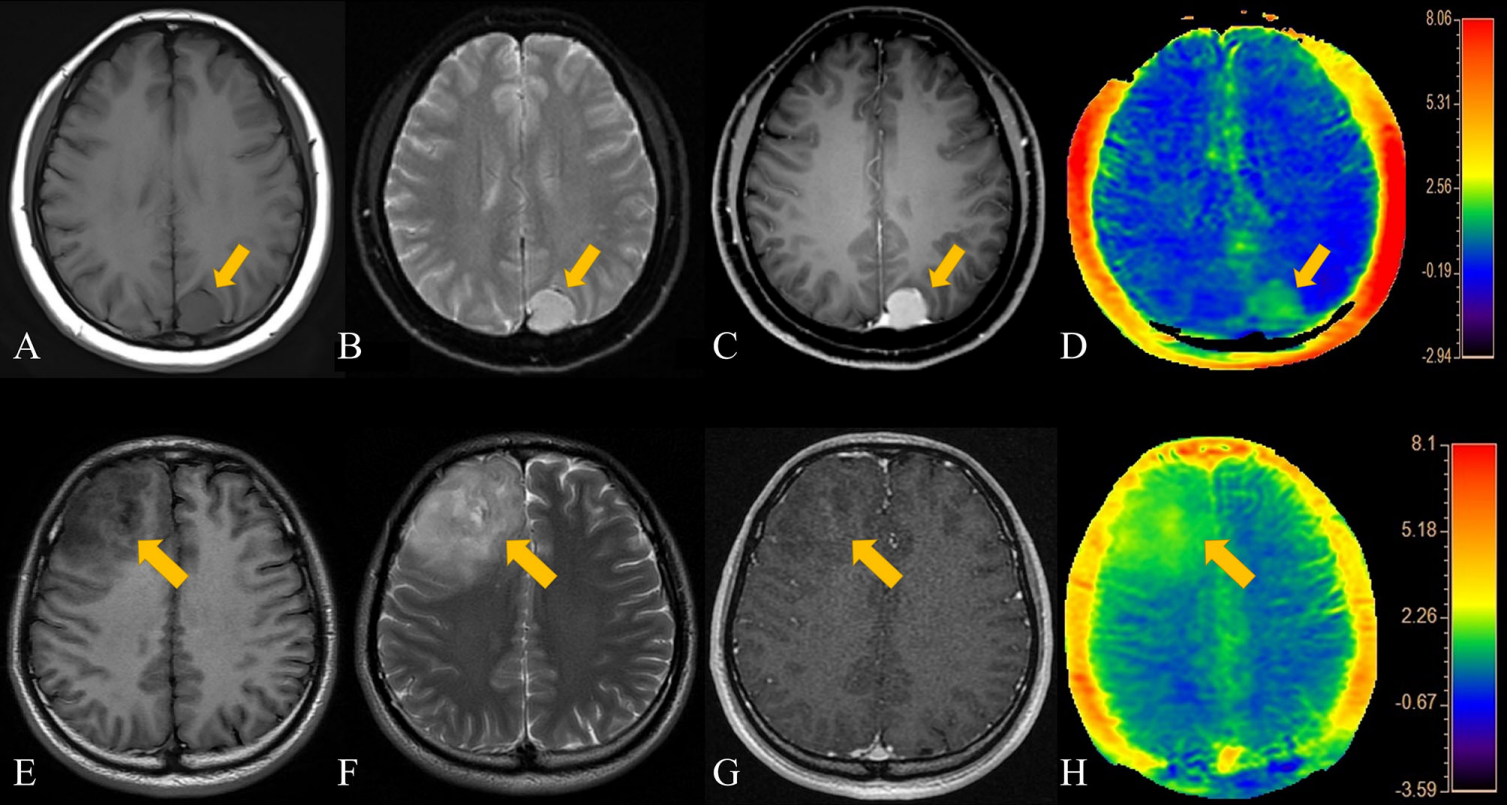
Patient 1, a 44-year-old female, presented with a left parietal lobe meningioma. The patient's T2WI, contrast-enhanced MRI, and APT range were similar. (A) T1-weighted imaging showed an iso-slightly low signal. (B) T2-weighted imaging showed an iso-slightly high signal. (C) Enhanced MR imaging showed marked enhancement. (D) APT imaging shows a slightly higher rate of amide proton transfer. Patient 2, a 34-year- old male, had an LGG in the right frontal lobe. The APT signal range of the patient was slightly larger than that on both the T2WI and enhanced scans. (E) T1-weighted imaging showed an iso-slightly low signal. (F) T2-weighted imaging showed an iso-slightly high signal. (G) Enhanced MRI showed mild enhancement. (H) APT imaging showed that the amide proton transfer rate slightly increased.
